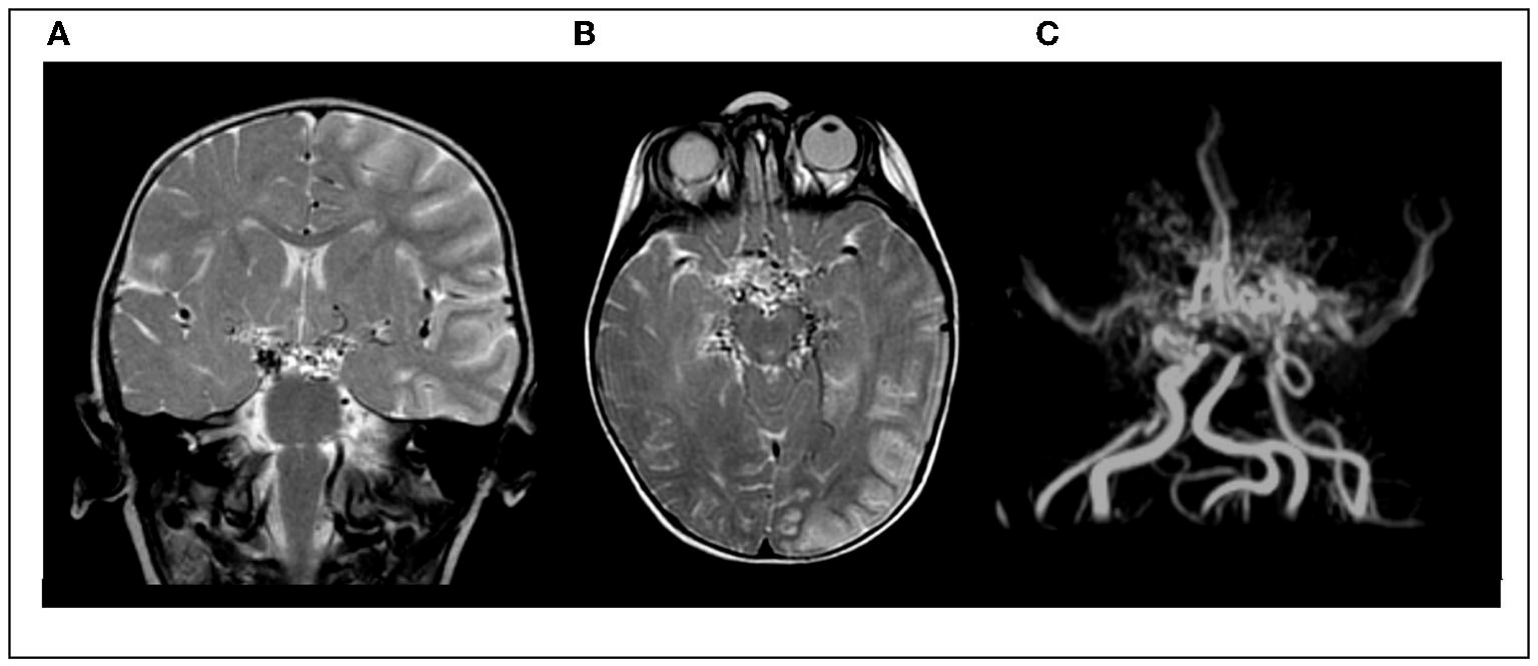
Cerebral MRI and MRA study in an 11-month-old girl with early-onset MMD, presenting with acute ischemic stroke. (A,B) Coronal and axial T2-w image showing left-sided cortical and subcortical infarct; multiple bilateral “flow voids” in the basal ganglia. (C) Magnetic resonance angiography showing typical “puff of smoke”.
